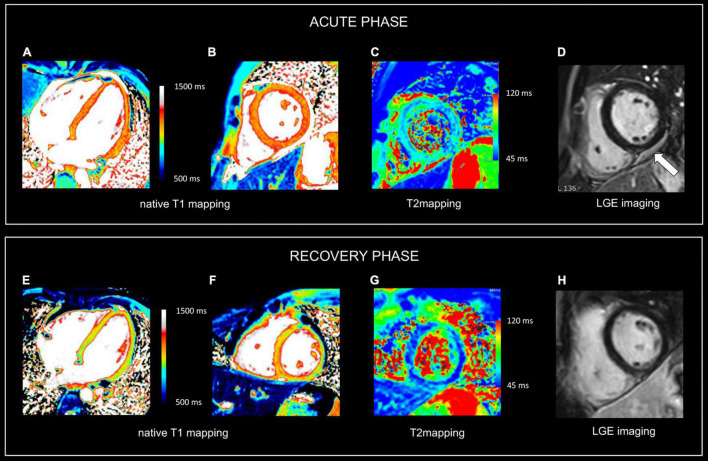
Use of CMR in acute and follow-up phase of diffuse myocarditis. Sixteen-year-old boy admitted with viral myocarditis and heart failure. Increased troponin I (12,239 ng/L), NTproBNP (23,731 ng/L) and c-reactive protein (183 mg/L). At admission, transthoracic echocardiography shows non-dilated but severely dysfunctional LV (EF 20%) and mild pericardial effusion. CMR, performed five days later, shows almost complete functional recovery (i.e., LV ejection fraction 57%). Although LGE imaging (D) shows no focal myocardial abnormalities, native T1, T2 and ECV values are diffusely increased, i.e., 1125 ms, 69 ms and 34% [panels (A–C), respectively]. Presence of limited pericardial effusion [arrow, (D)]. Repeat CMR 4 months later (recovery phase) shows normalization of T1, T2 and ECV values, i.e., 975 ms, 51 ms, and 24% [panels (E–G), respectively], while LGE imaging (H) yields no evidence of permanent irreversible damage (i.e., focal replacement fibrosis). Moreover, a further improvement in LVEF was noted, i.e., 64% with decrease in LV mass (i.e., 83 vs. 102 g at baseline), and disappearance of the pericardial effusion. In the clinical setting, the baseline CMR findings most likely represent diffuse myocardial edema/inflammation caused by viral myocarditis. As the myocardium is diffusely affected, weighted sequences, i.e., T2 and LGE imaging are “normal” emphasizing the added value of parametric mapping in these patients.
